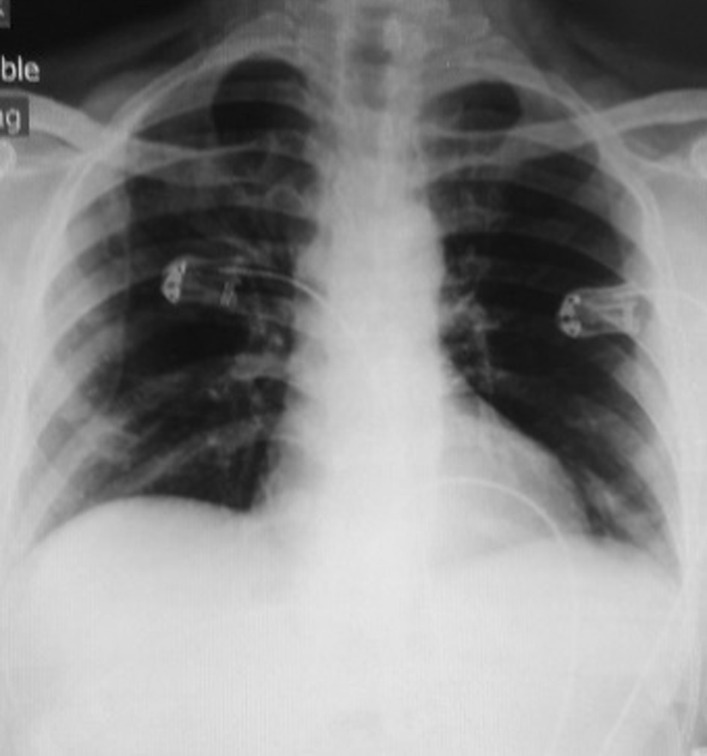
Initial chest X-ray, anterior–posterior view
